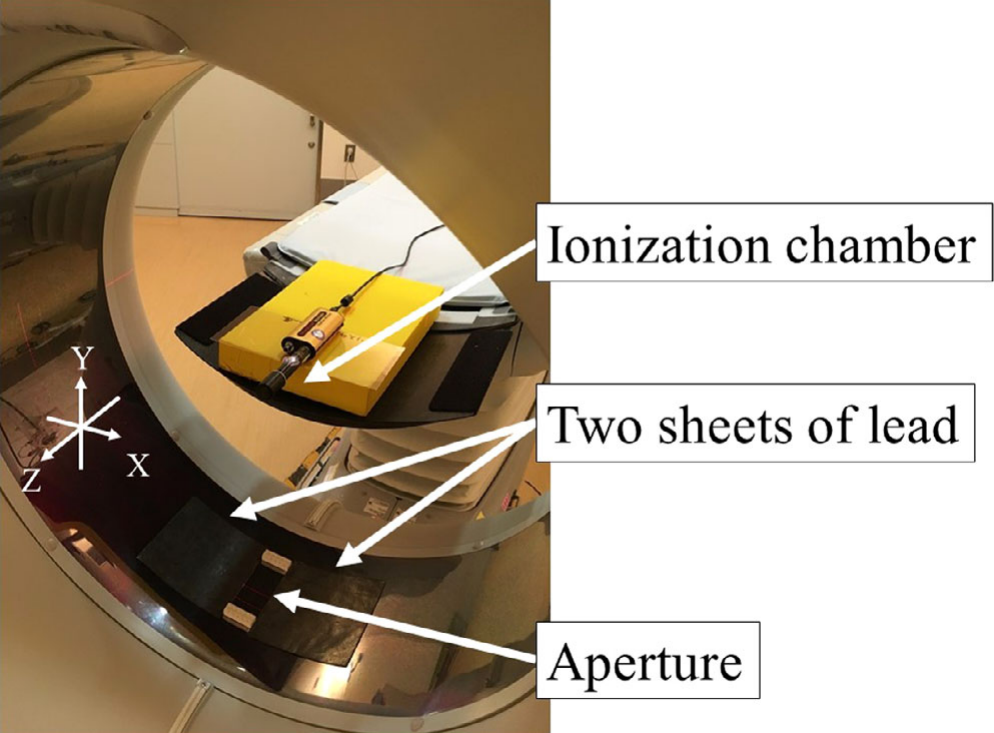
Experimental arrangement for measurement of the ${\dot{K}}_{\text{w}/\text{o}-\text{A}}$ and ${\dot{K}}_{\text{w}-\text{A}}$. A 6‐cc ionization chamber was suspended free‐in‐air at the isocenter of the CT system, and two sheets of lead (160 mm × 160 mm × 1 mm) were placed on the bottom of the gantry cover to form apertures (40, 48, 56, 64, 72, and 80 mm). The apertures were set to collimate the radiation beam along the x‐axis.
